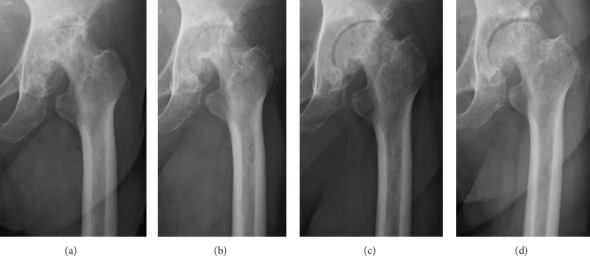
Plain radiograph for case 2, showing the hips at (a) first admission and at (b) 1 year, (c) 2 years, and (d) 3 years after the initiation of jiggling exercise.
